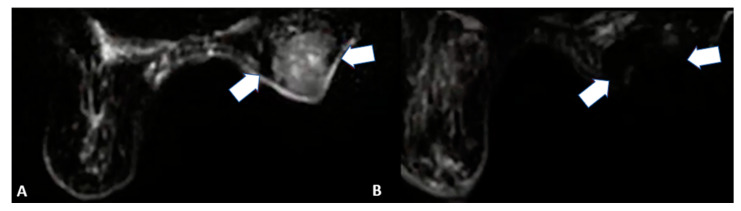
85-year-old female patient with invasive right breast cancer with axillary metastases. Diffusion-weighted imaging MRI scan showing (A) baseline large neoplastic lesion infiltrating the skin and soft tissues (arrows) and (B) 4-month follow-up after microwave ablation with absent signal restriction (arrows).
